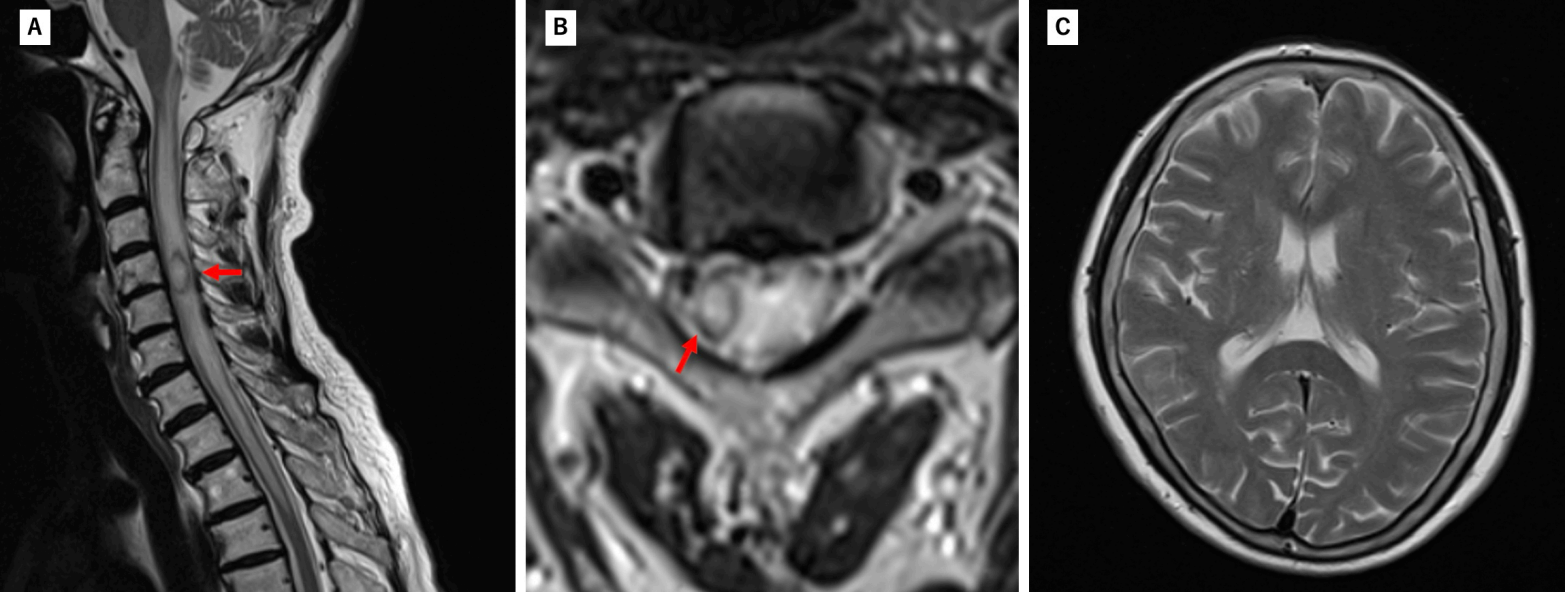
MRI on admission revealed a hyperintense intramedullary lesion (red arrow) on the right side at the C4-C5 spinal level with extensive perilesional edema extending to the brainstem. Brain MRI showed no obvious abnormalities. (A: T2WI Sagittal; B, C: T2WI Axial). MRI: magnetic resonance imaging; T2WI: T2 weighted image.
